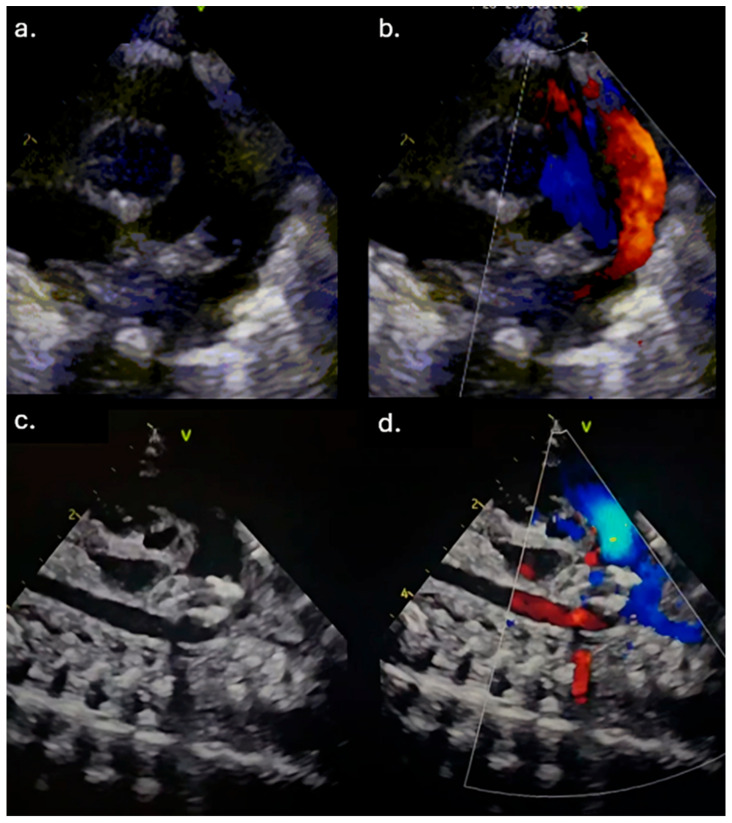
(a) Echocardiography examination before patent ductus arteriosus (PDA) closure. (b) Moderate left-to-right shunt across PDA. (c,d) Echocardiography pointed out the intraductal position of the Amplatzer Piccolo Occluder.
